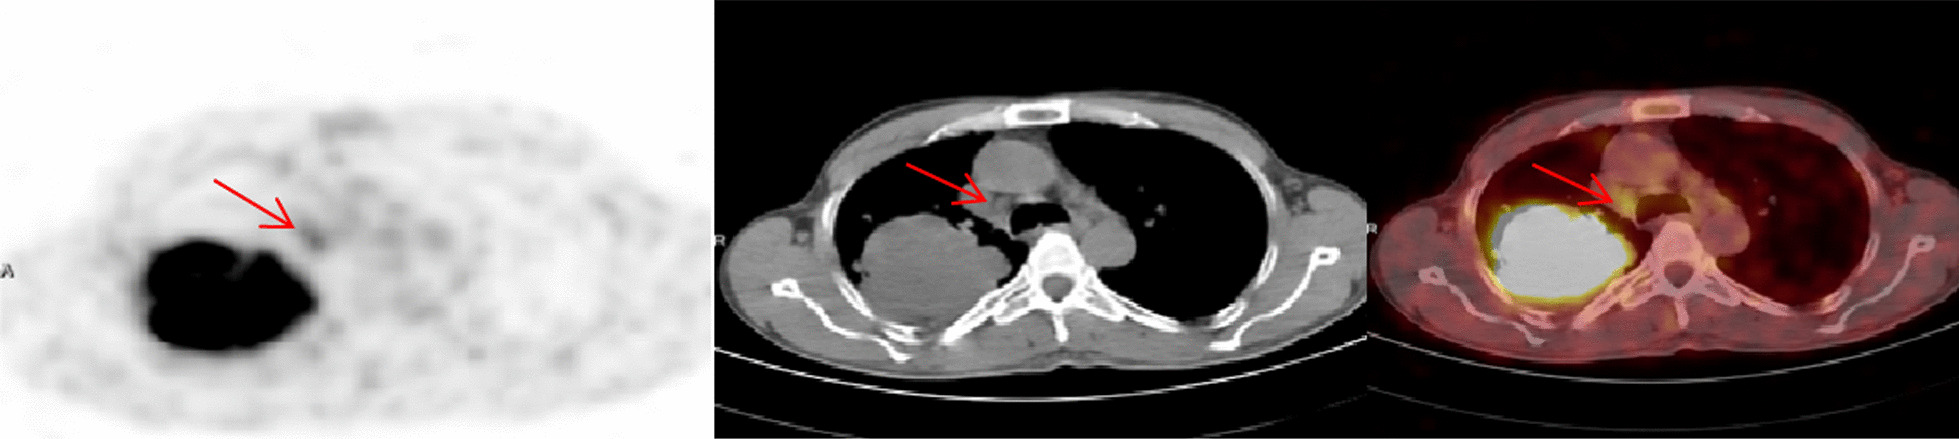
True positive FDG-PET/CT and false negative EBUS/TBNA. A case of SCC involving the right lung. Axial FDG-PET, CT and FDG-PET/CT images showed a suspected prominent hypermetabolic (SUVmax: 3.1) right paratracheal lymph node (arrows) and recommended tissue confirmation. EBUS/TBNA turned out negative; Mediastinoscopy proved a metastatic lymph node
